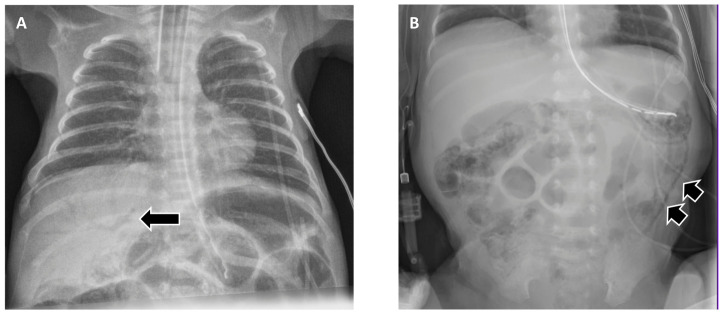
Classic abdominal radiographic findings in necrotizing enterocolitis. (A) Supine abdominal radiograph demonstrating portal venous gas (black arrow), seen as branching, linear lucencies within the hepatic parenchyma, reflecting intravascular gas within the portal venous system. (B) Supine abdominal radiograph demonstrating pneumatosis intestinalis, with linear “train-track” intramural lucencies along the bowel wall (arrowhead). These radiographic findings typically reflect mucosal injury with translocation of gas-producing bacteria into the bowel wall and portal venous system and are associated with advanced intestinal injury in NEC.
